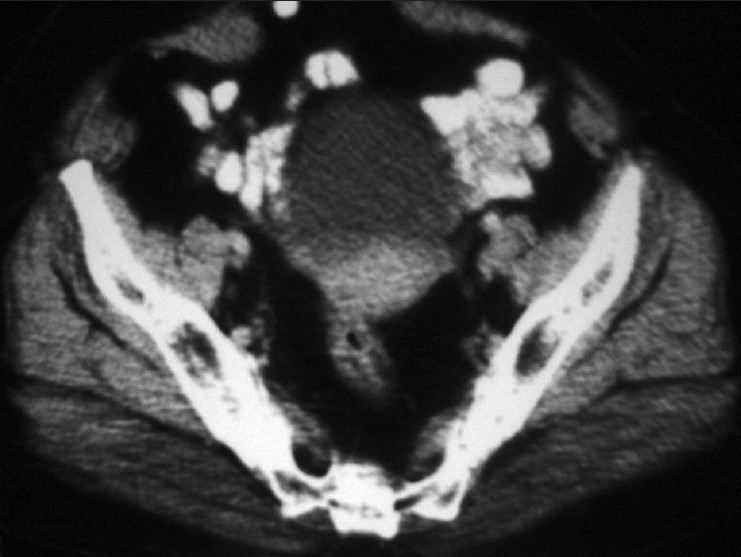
Contrast-enhanced computed tomography scan of the abdomen (post-treatment) revealed no obvious adenexal lesion in this scan, which was present on the pre-treatment scan
